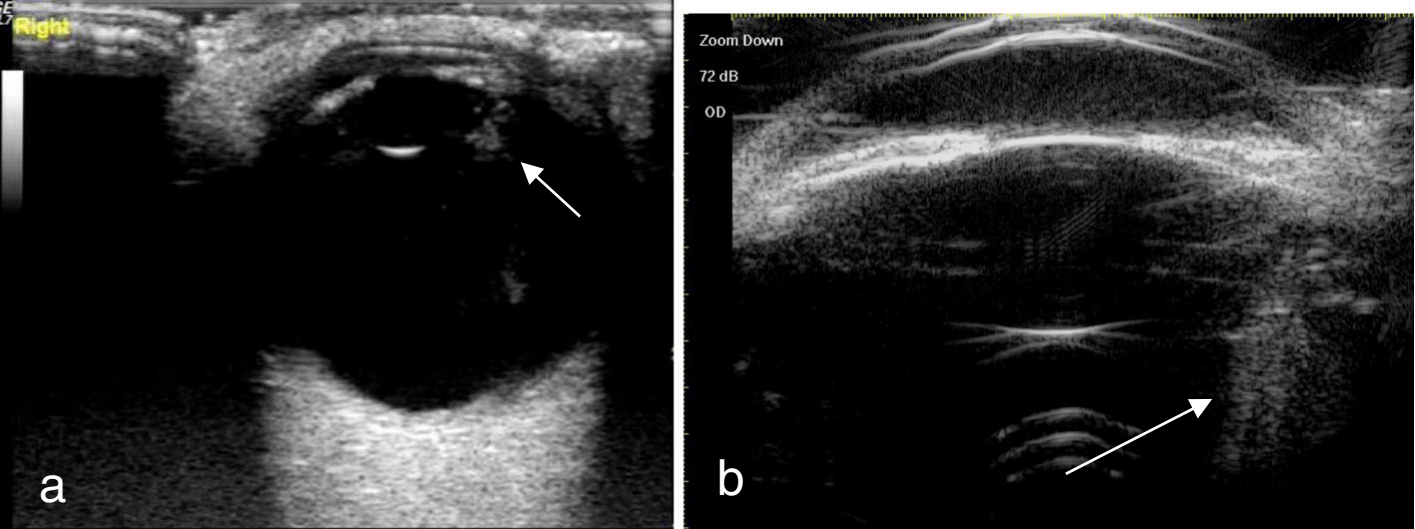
a The false positive image taken with 14-MHz + THI. A moderate echo mass is seen in the equatorial part of the lens, which is mistaken for a PC rupture and cortex overflow (short arrow). b: False positive LFUBM image. The ultrasonic performance is similar to that shown in a (long arrow)
